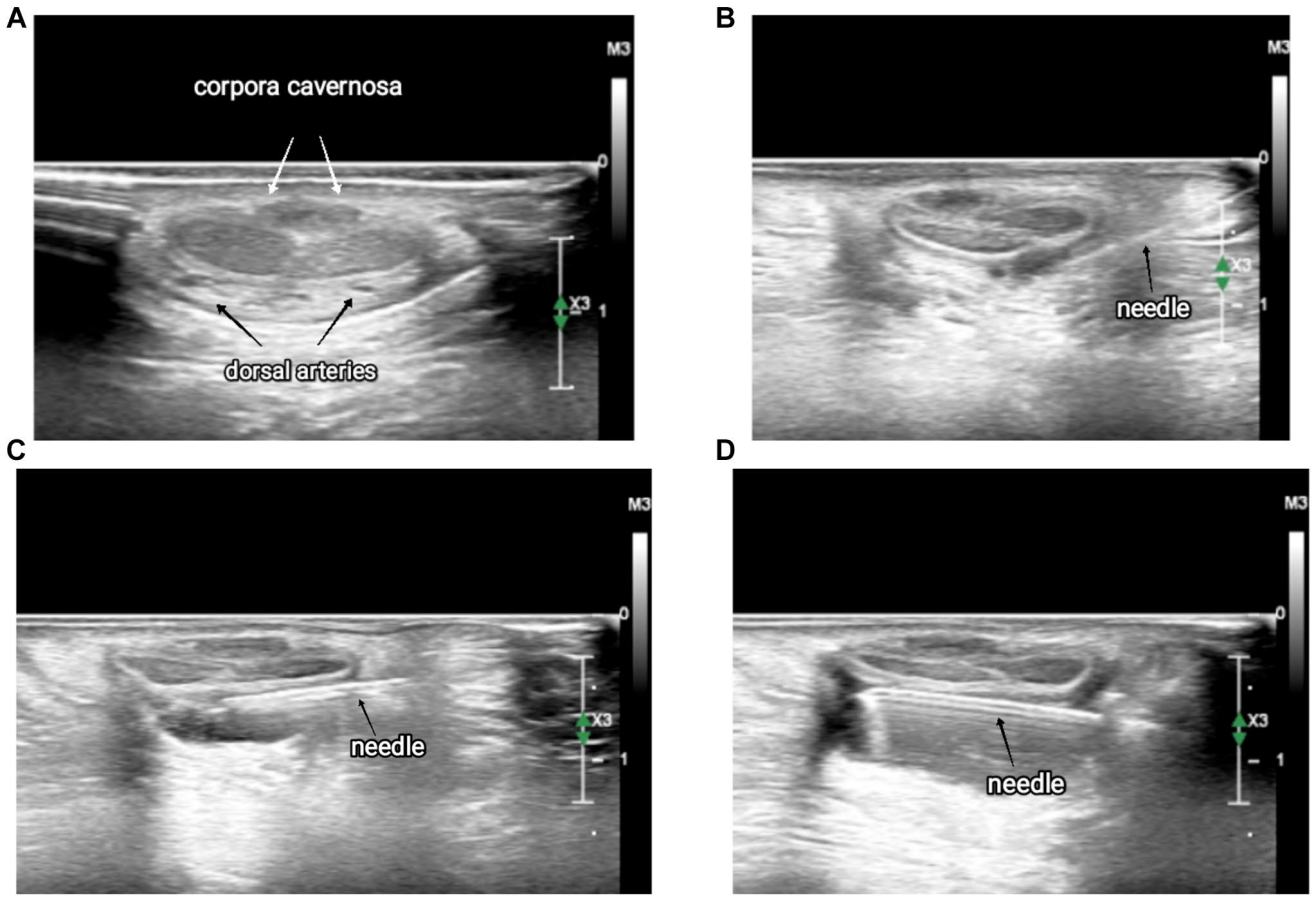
Sequence of ultrasound images of the dorsal penile nerve block. (A) Anatomy of the penis, (B) local anesthetic injection on the right-hand side, (C) local anesthetic injection in the median region, and (D) local anesthetic injection on the left-hand side.
